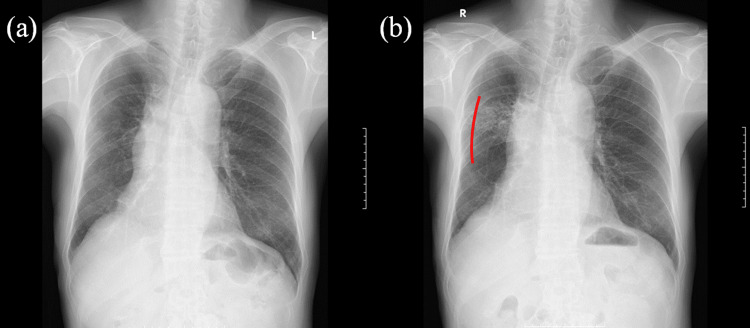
X-ray photographs X-ray photograph (a) on the day the CBCT image suggested RP at a cumulative dose of 50 Gy, and X-ray (b) three weeks later showing RP indicated with a red line. Radiotherapy was continued to a full dose of 60 Gy due to a strong request from the patient, with oral prednisolone administration. CBCT - cone-beam computed tomography; RP - radiation pneumonitis
